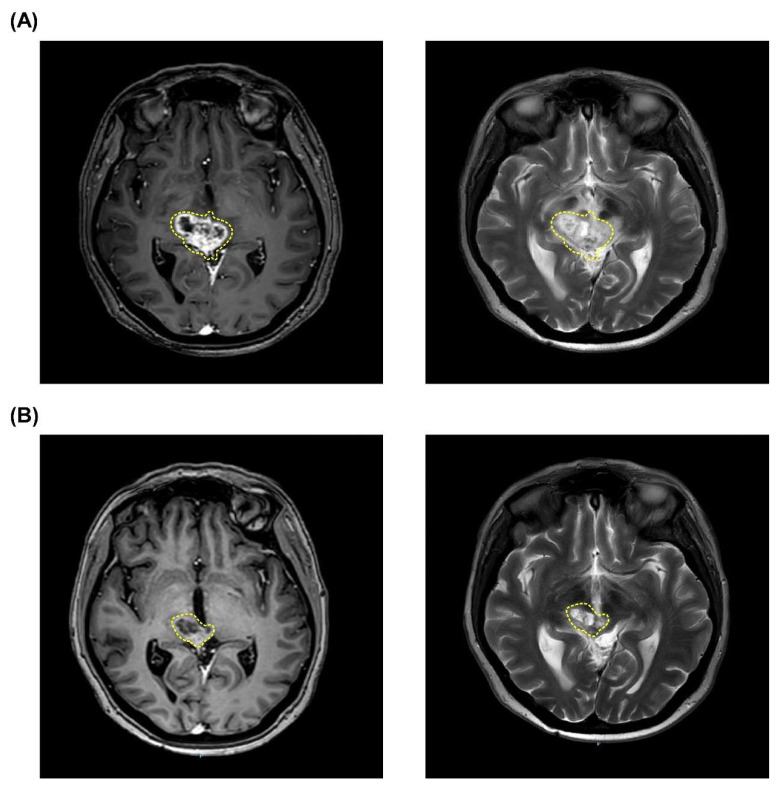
MRI images of tumor shrinkage of Case 2 (patient #3). Tumor volume before (A) and after (B) a scheduled two fractions of salvage BNCT procedures. The pleomorphic xanthoastrocytoma in the images is indicated by yellow dashed circles.
